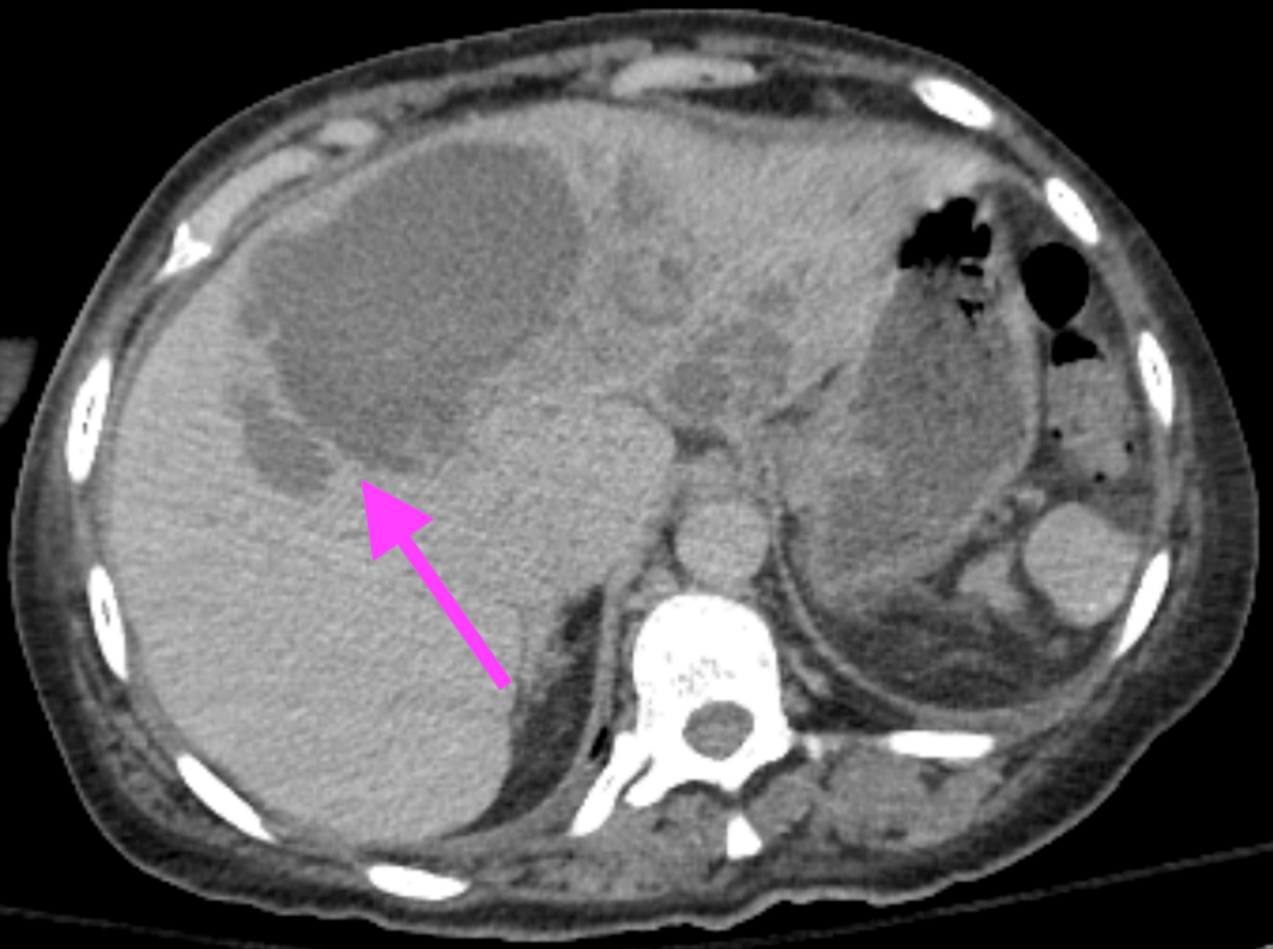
A CT scan of the abdomen and pelvis demonstrated a multiseptated hypodense collection within the liver, indicating an abscess with cholelithiasis. The arrow highlights the intrahepatic abscess.
